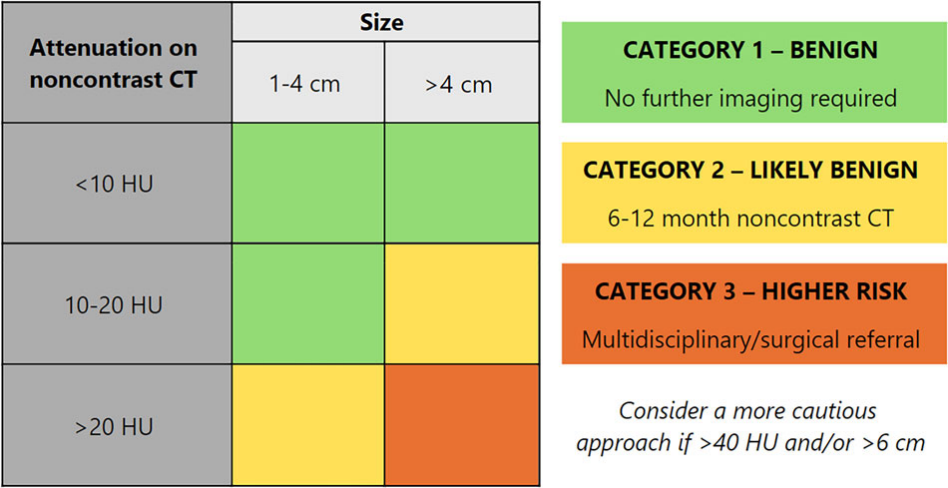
Management of adrenal incidentalomas on noncontrast CT^a^. ^a^ Table only to be used in conjunction with summarised recommendations. HU, Hounsfield unit
